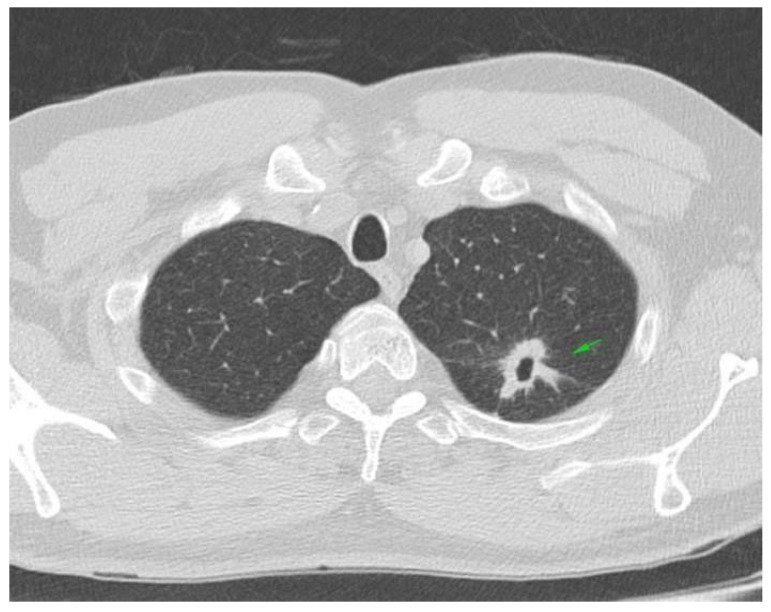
Chest CT scan: left superior lung lobe tumor cT2cN0.
